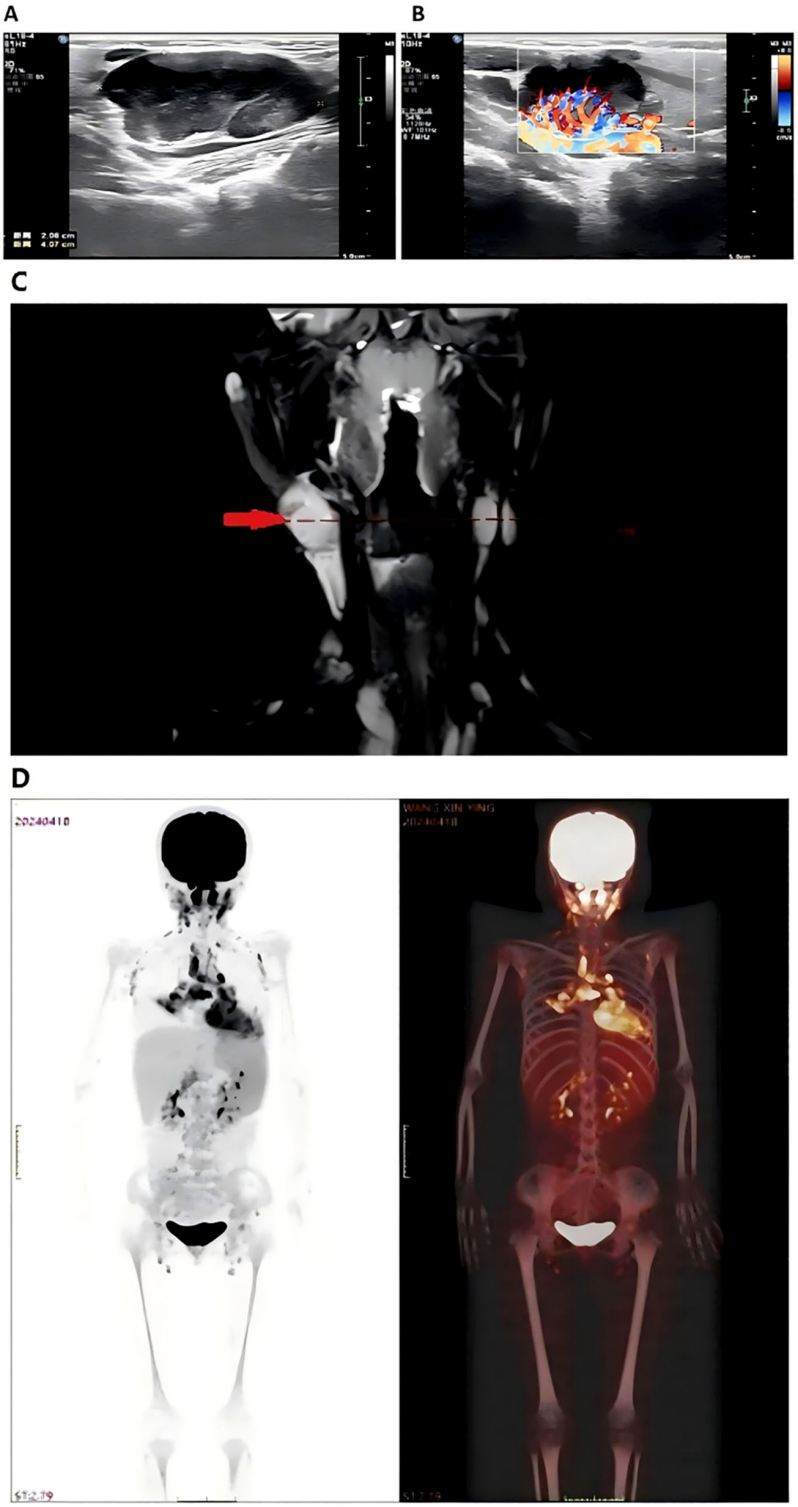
Imaging of bilateral parotid and neck regions showed a hypoechoic mass measuring 4.1×2.8×2.1cm (A) surrounded by hypoechoic nodules with abundant blood flow on Doppler imaging (B) Neck MRI (C) displayed abnormal signals in the right nasopharynx with multiple enlarged lymph nodes, raising suspicion for Hodgkin lymphoma or nasopharyngeal carcinoma. Whole body PET-CT scan (D) revealed increased metabolic activity in neck, mediastinal, and abdominal lymph nodes, consistent with lymph node involvement. Splenomegaly and heightened FDG uptake in the thymus were also noted.
